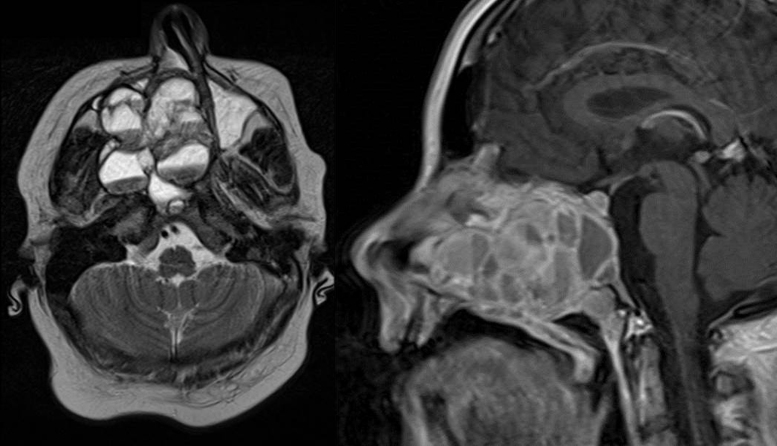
MRI of the head. Axial T1 weighted image (left) and sagittal T1 weighted image (right): an extensive iso- to hypertense lesion of the midface with multilocular conspicuous fluid levels, enhancing septations and solid components. Tumor affected the medial, lateral and ventral wall of the right maxillary sinus. Contralateral it destructed the medial wall of the left maxillary sinus. Erosions of the right bony palate and of major parts of the dorsal septum and the inferior concha nasalis on both sides were observed. The dorsal expansion leads to the clivius, which was partly destructed, and to destruction of the pterygoideus process on the right. Radiologic investigations furthermore revealed partly destruction of the medial wall of the internal carotic artery and partly destruction of the orbital floor and lamina papyracea right.
